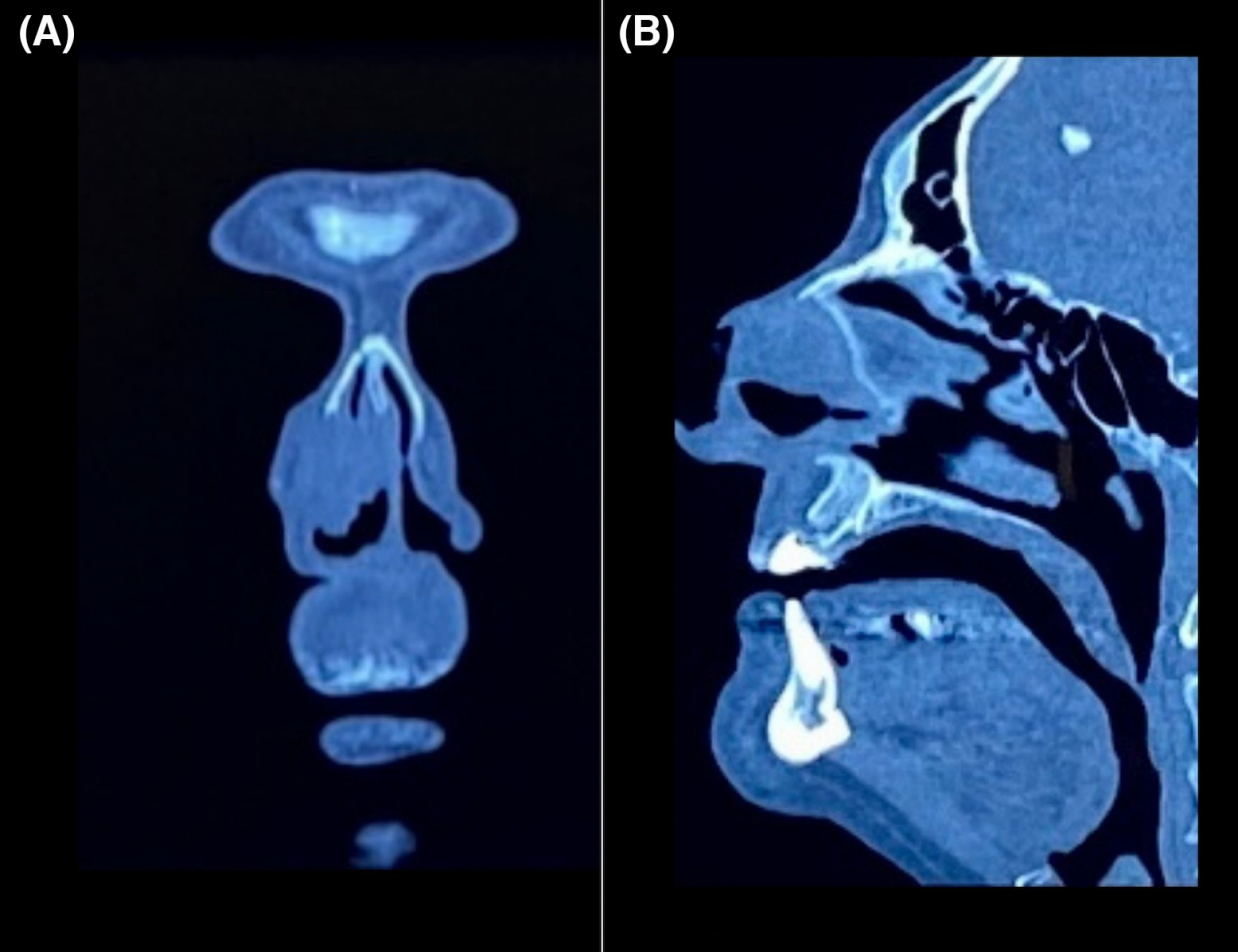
(A) Bone window tomography on coronal section: right nostril is occupied on 80% by a tumor that is in intimate contact with the septum on its cartilaginous portion with no bone erosion. (B) Bone window CT on sagittal section: tumor is seen in the right nostril in contact with the cartilaginous septum, no bone erosion is observed and the nasal bones are spared. No involvement of the paranasal sinuses and skull
